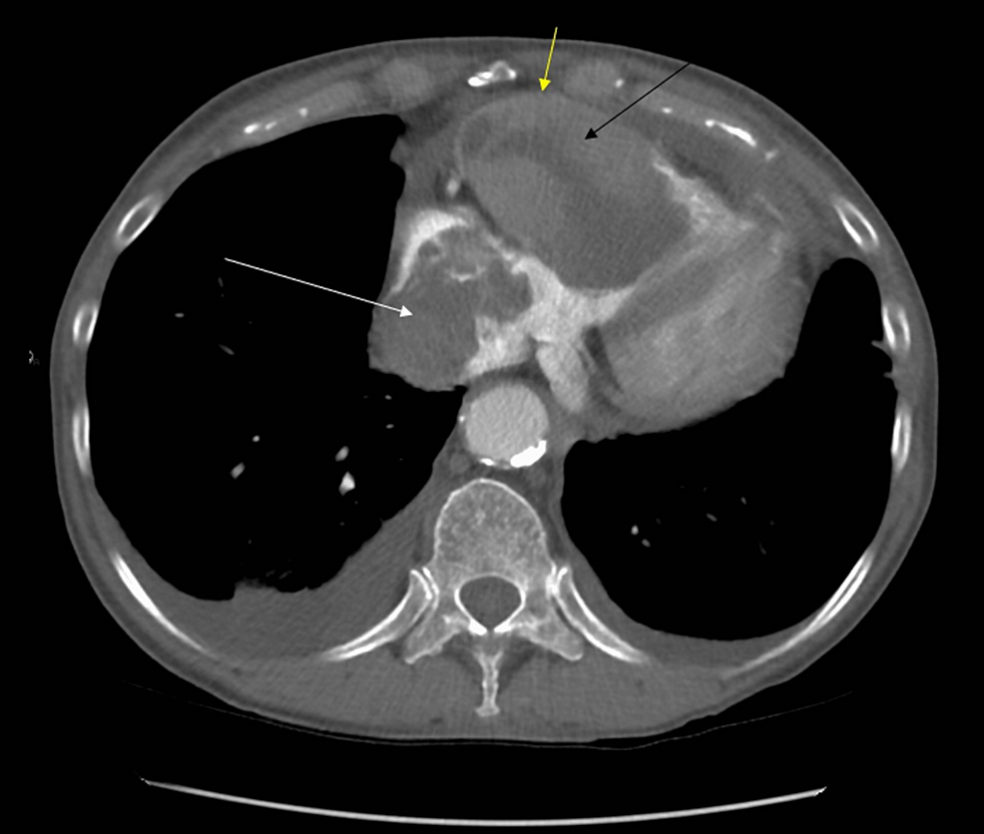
CT chest with contrast in the transverse plane. There is a large mass of mixed attenuation within the right ventricle (black arrow). There is an extension of the mass into the right atrium that also contains a mixed attenuation with likely thrombus formation (white arrow). There is no contrast separating the ventricular mass from the wall, likely indicating invasion or attachment to the ventricular wall (yellow arrow).
